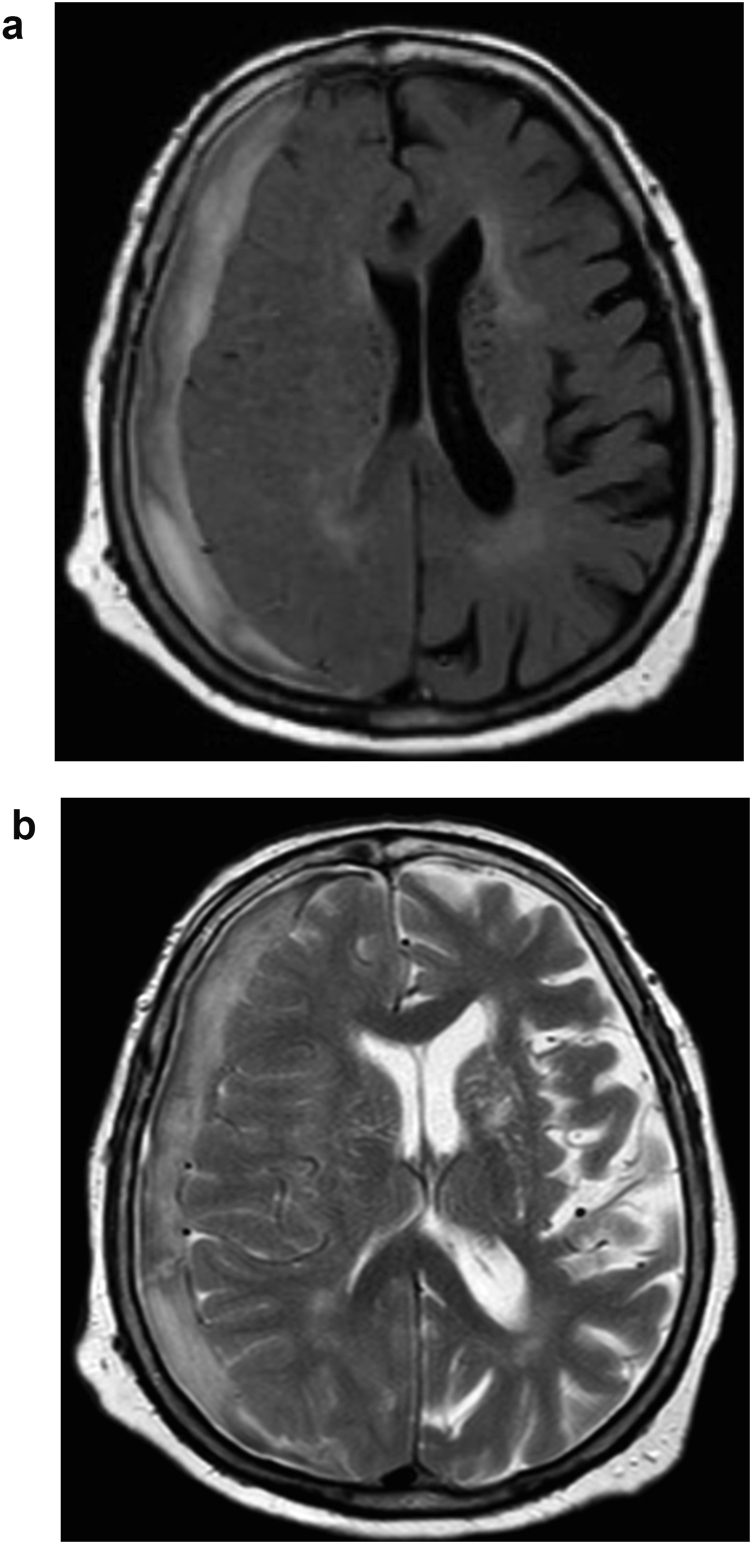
Brain MRI showing hyperintensity rim in T1W (a) and iso-to hypo-intensity in T2W (b) compatible with early subacute subdural haematoma.
